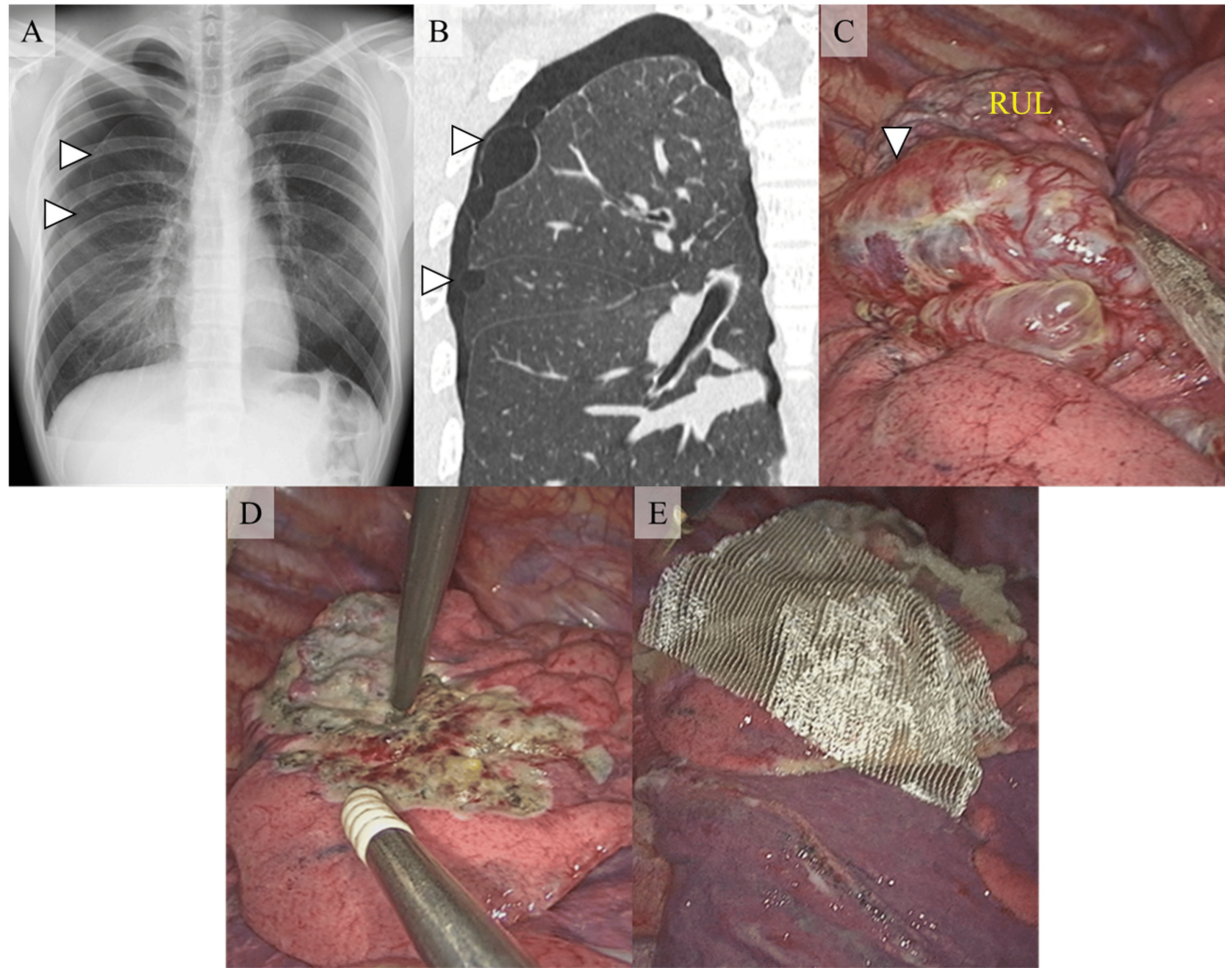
Radiological images and intraoperative findings of first surgical treatment A: Chest X-ray revealed right-side spontaneous pneumothorax; B: Chest computed tomography showed 7cm bulla in the right upper lobe and 1cm bulla in the right middle lobe; C: A large bulla arises from the cranial to the caudal part of the right upper lobe; D: Cautery ablation with soft coagulation of each bulla was performed; E: Almost the whole area of the right upper lobe is covered with ORC mesh sheets. ORC: Oxidized regenerated cellulose; RUL: Right upper lobe
